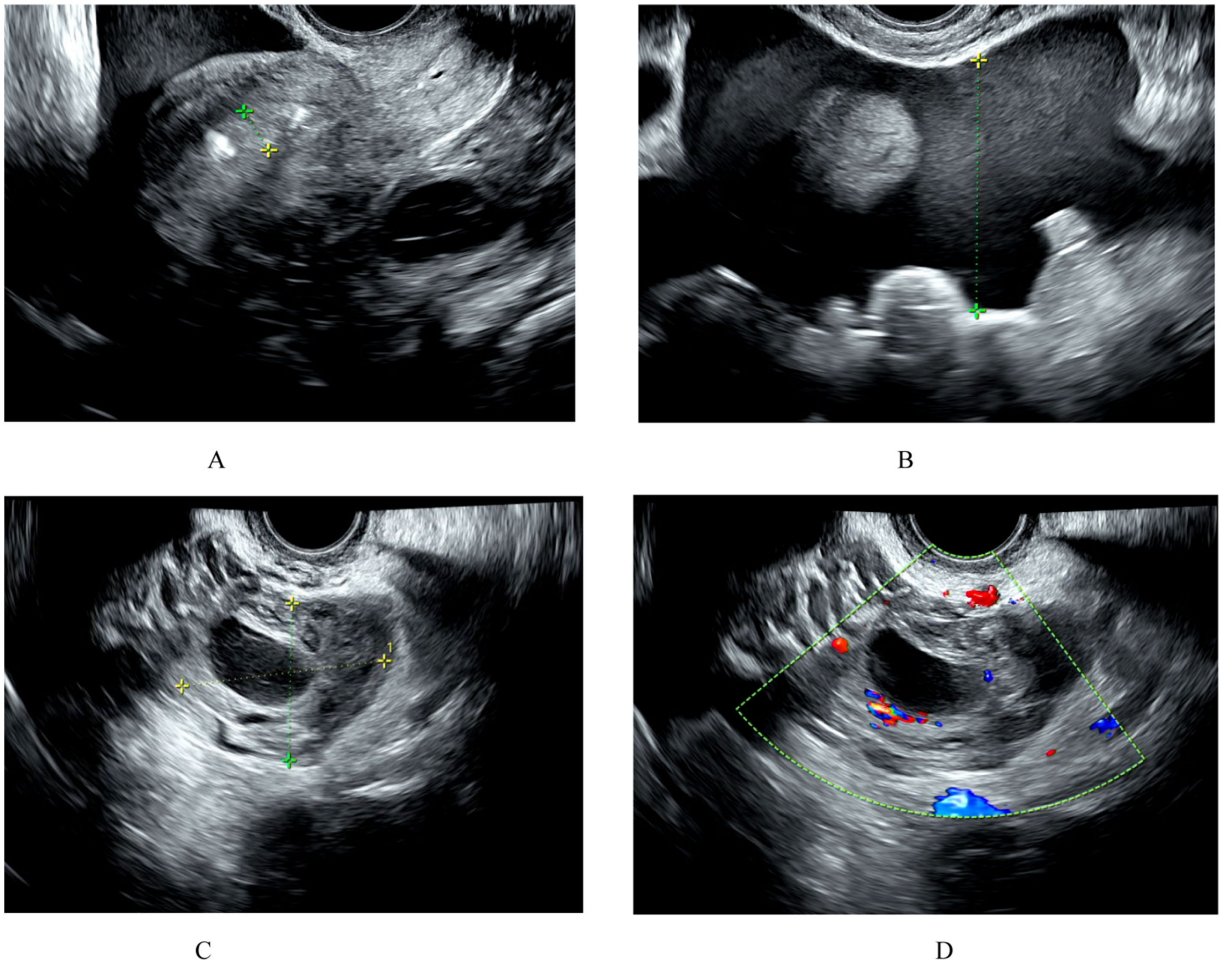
(A) The endometrium is thickened to 1.1 cm. An IUD is visible in the uterus, and its position is correct. No gestational sac is seen in the uterus. (B) Hemorrhagic fluid is visible in the pelvic cavity. (C,D) A mixed echo of 5.1 × 3.6 cm is seen in the left adnexal region, and ectopic pregnancy is suspected on ultrasound.
